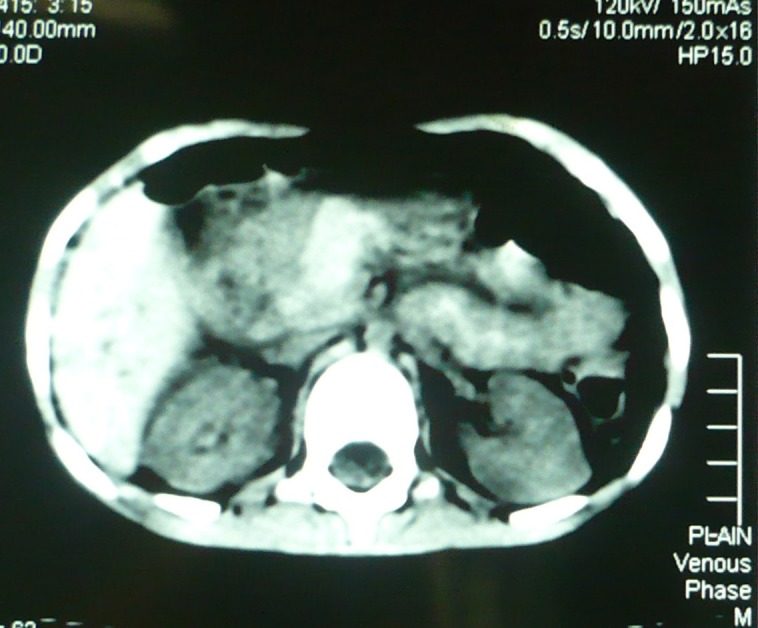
Figure 1: CT scan of abdomen showing pancreatic contusion injury; the pancreas is swollen and duodenal area is obscured by hypodense area.
